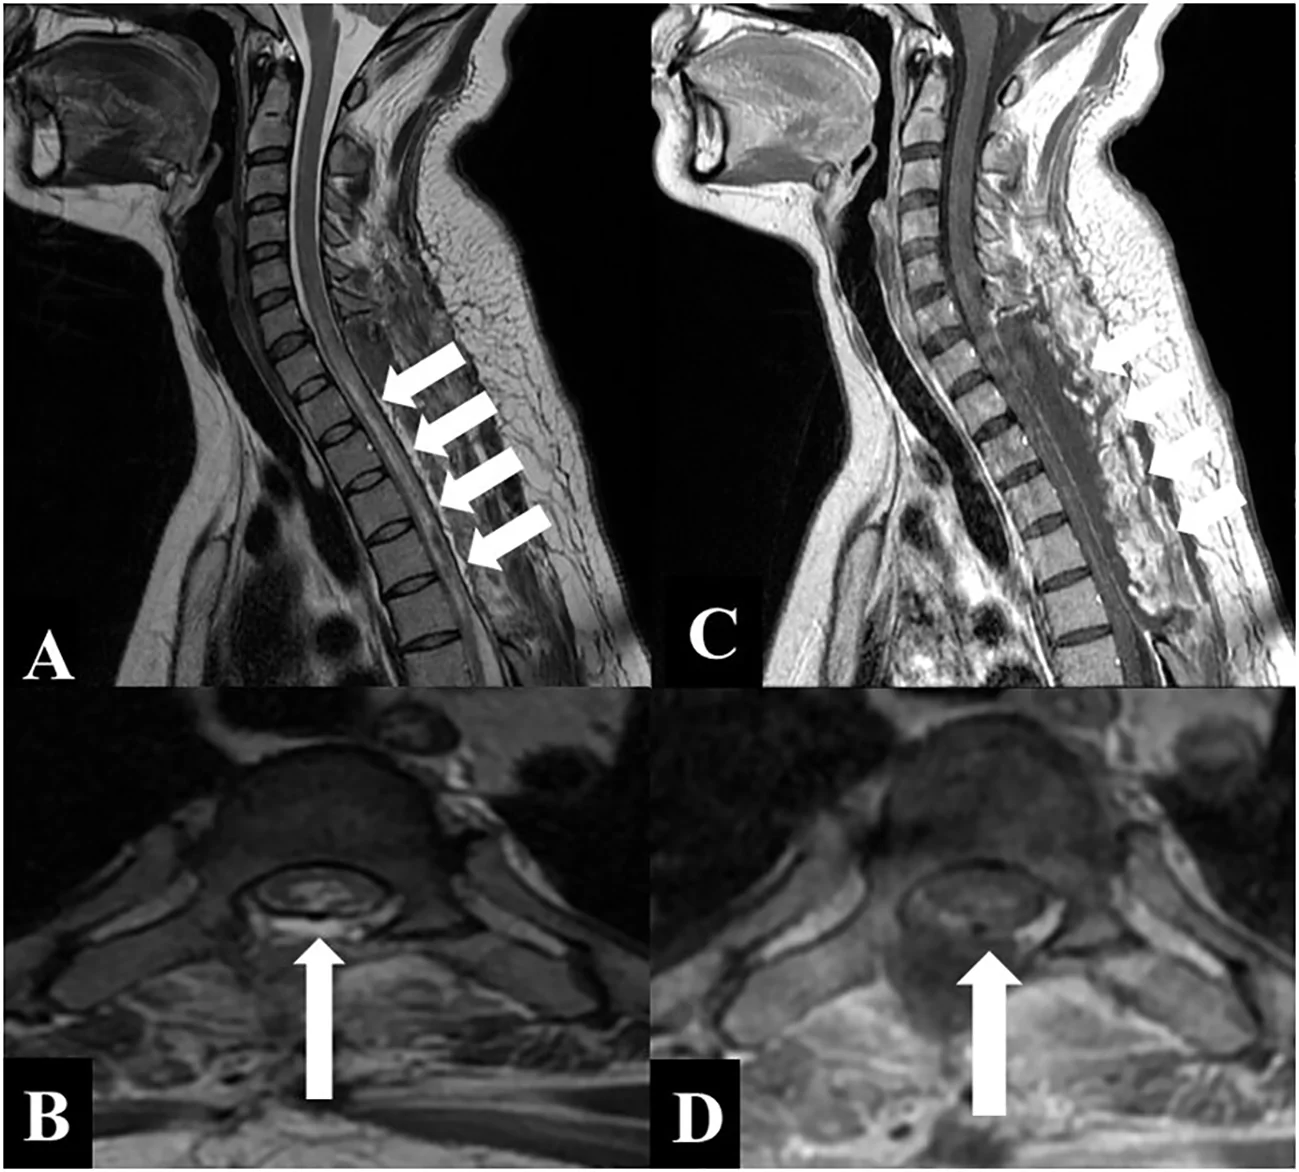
Postoperative 1-day magnetic resonance imaging of the cervicothoracic spinal cord. (A) Sagittal T2-weighted image; (B) Axial T2-weighted image; (C) Sagittal contrast-enhanced T1-weighted image; (D) Axial contrast-enhanced T1-weighted image. Images demonstrate postoperative changes following gross total resection, including a postoperative CSF-filled resection cavity without evidence of residual enhancing tumour. White arrows indicate the postoperative resection cavity (site of tumour removal).
